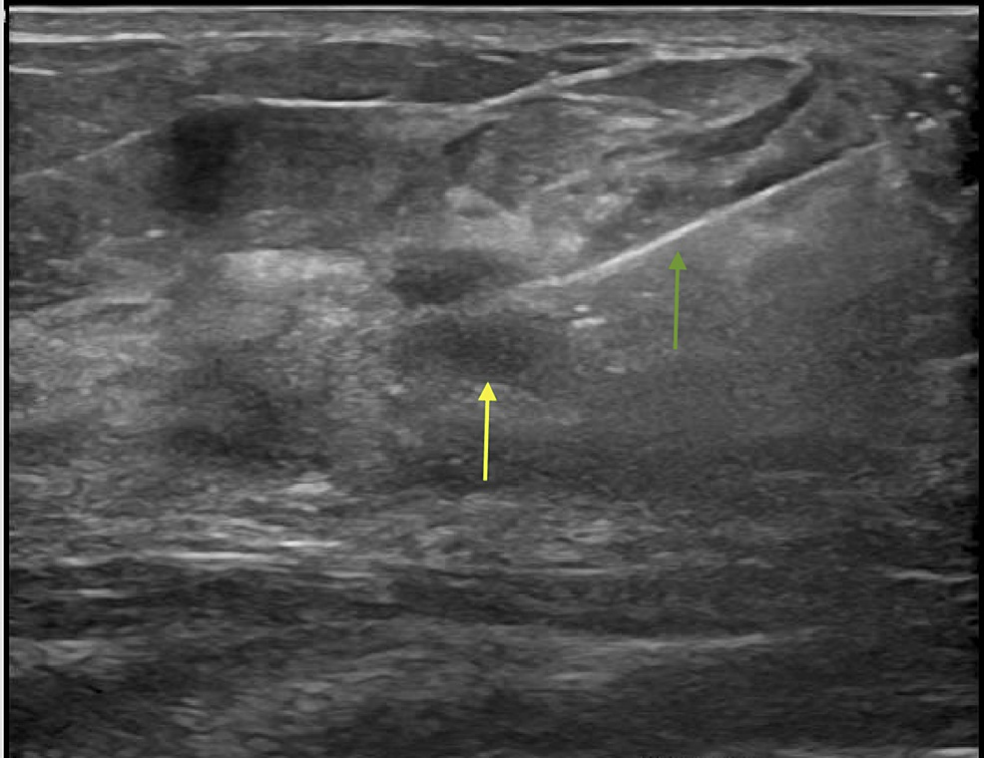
Ultrasound-guided biopsy of the 12 o'clock mass Ultrasound findings stated that "biopsy of the 1.2 cm mass in the right breast at 12 o'clock anterior depth was successful. Pathology indicates adenoid cystic carcinoma. A mass demonstrated at 11 and one o'clock anteriorly on MRI could not be found by US." Green arrow: Biopsy needle Yellow arrow: Mass
